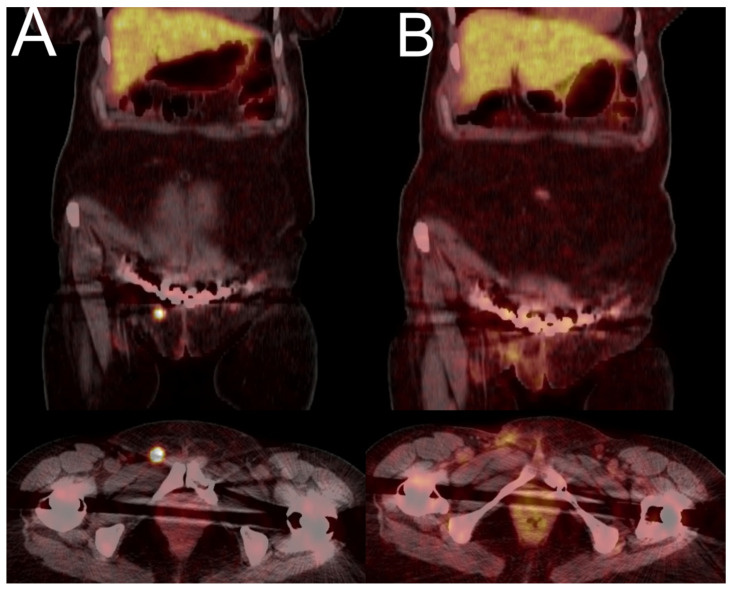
[^68^Ga]Ga-DOTATATE PET/CT images of a 58-year-old woman with a near 4-year history of osteoporosis and hypophosphataemia (Case 3). The [^68^Ga]Ga-DOTATATE PET/CT images show intense focal uptake in the soft tissue of the pubic region with an SUVmax of 40.8 (A). After surgery, phosphatase slightly increased. The follow-up [^68^Ga]Ga-DOTATATE revealed a focal recurrence with an SUVmax of 2.6 (B). Upper row—fused frontal PET/CT images, lower row—fused transaxial PET/CT images of the pelvis.
